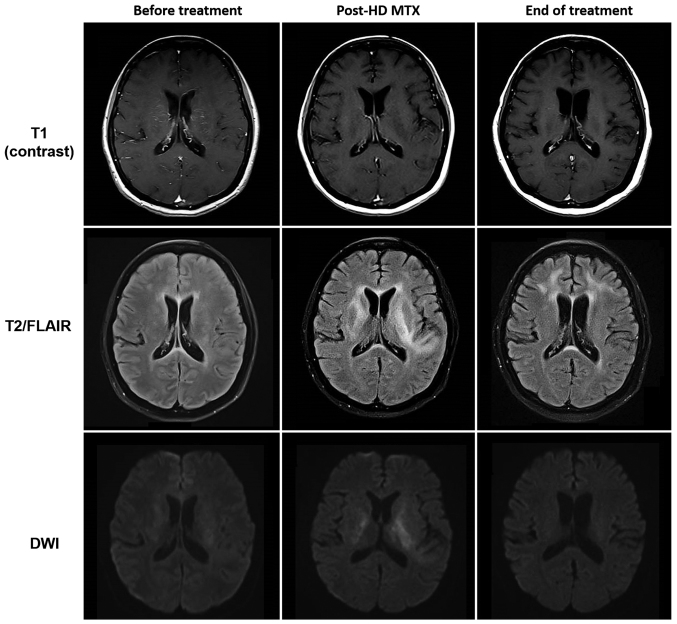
Reversible methotrexate leukoencephalopathy in 46-year-old female patient who presented with seizure, choreoathetosis and altered mental status. HD MTX, high-dose methotrexate; FLAIR, fluid-attenuated inversion recovery; DWI, diffusion-weighted imaging.
